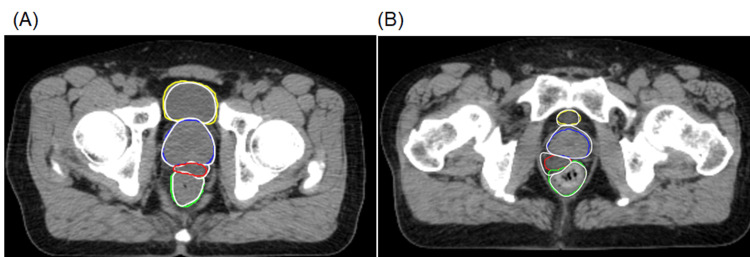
CT-based automatic and reference contours Representative axial planning CT images showing CT-based automatic contours (OncoStudio; colored lines) overlaid with reference contours (white dashed lines) for the rectal spacer (red), prostate (blue), rectum (green), and bladder (yellow). Panel A shows a case with high agreement, in which the automatic and reference spacer contours closely overlap in the retroprostatic space. Panel B shows a case with a larger discrepancy in the spacer region.
